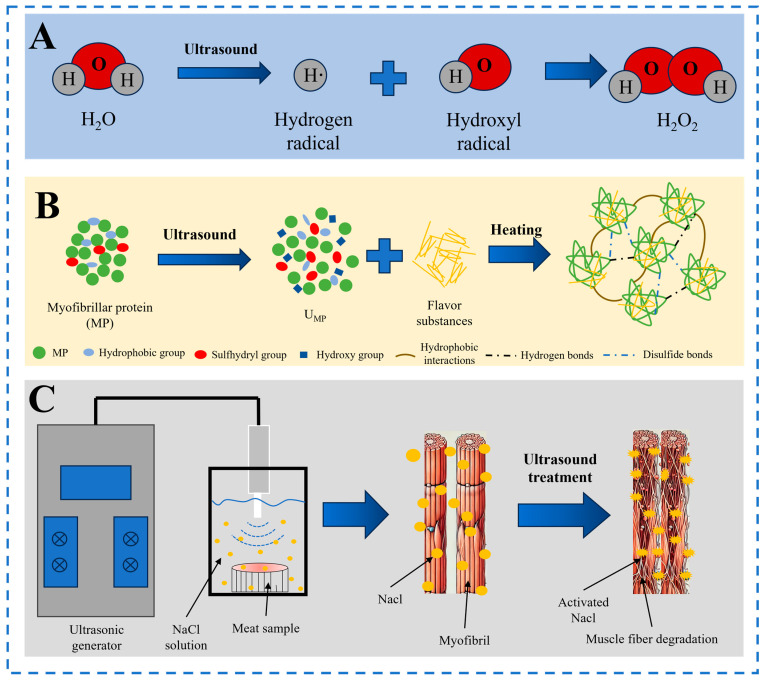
Water molecules are decomposed into highly reactive free radicals after ultrasound treatment (A). Ultrasound promotes the production of volatile flavor compounds (B). Ultrasound disrupts the structure of myofibrils and activates the brine, accelerating the penetration of sodium chloride (C).
